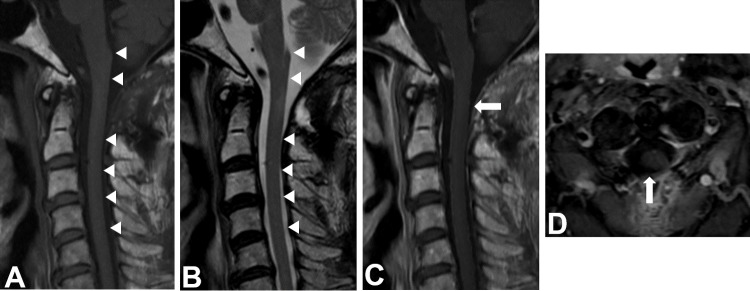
Postoperative magnetic resonance imaging Magnetic resonance imaging (MRI) performed three months after surgery showing no evidence of tumor recurrence and disappearance of the syringomyelia (A, B, C, and D) (arrow and arrowhead).
